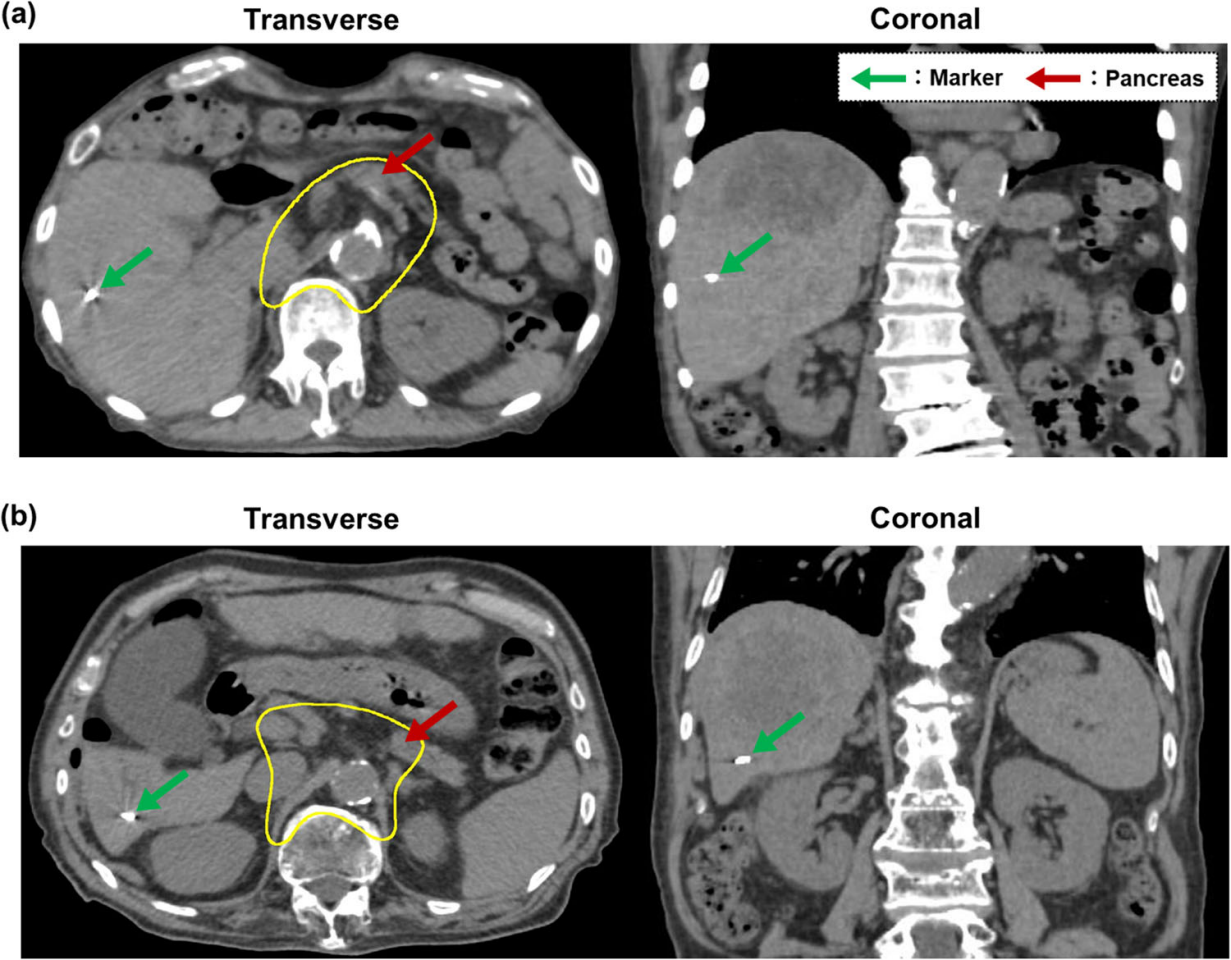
Location of the fiducial markers in the liver. Fiducial markers inserted in (a) S5 and (b) S6 are shown on the axial (left) and coronal (right) computed tomography (CT) images. Green and red arrows indicate the fiducial marker and pancreas, respectively. The yellow contour indicates the planning target volume (PTV).
